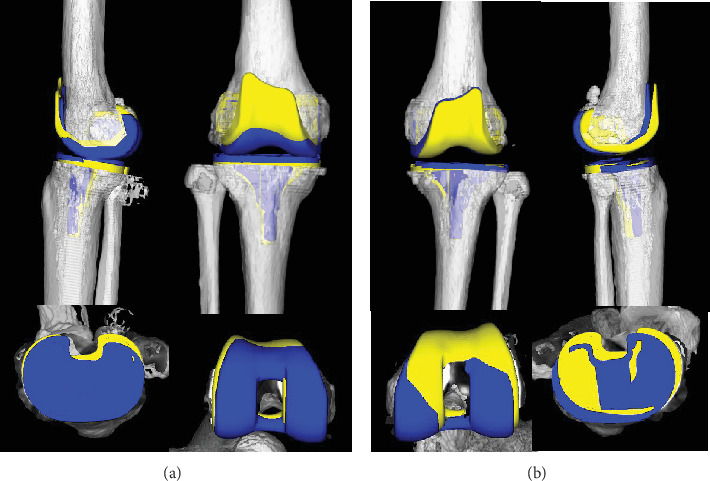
Preoperative three-dimensional computed tomography (3DCT) plan and postoperative 3DCT images were shown. 3D computer-aided design (CAD) data of femoral and tibial components were fitted to the 3DCT plan and images. Preoperative plan components are shown in blue. Postoperative components are shown in yellow. (a) Right knee and (b) left knee. The femoral component sagittal alignment in the left knee was 2° more flexed than the preoperative plan, but the right femoral component sagittal alignment in the right knee was similar to the preoperative plan.
